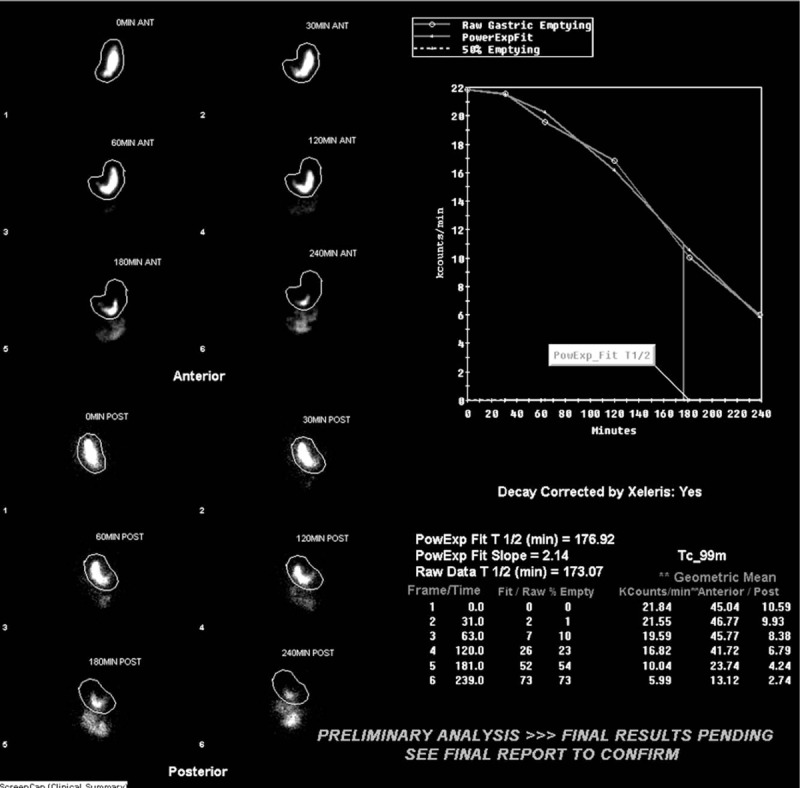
Delayed gastric emptying scintigraphy in a 55-year-old woman who underwent ReY. Pre-op GES: normal fundic accommodation; at 2 h remaining 77%, N < 60% and at 4 h remaining 27%, N < 10%. Post op: She complained of abdominal pain on short- and long-term follow-up.
